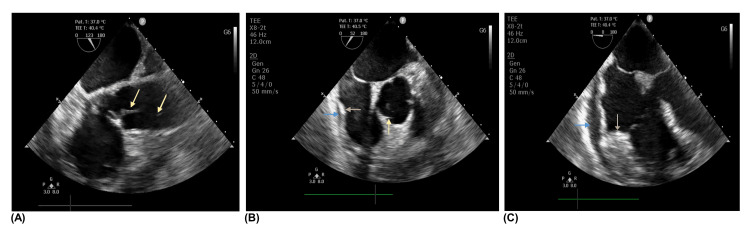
Complementary study during ICU stay - transesophageal echocardiogram. Transesophageal echocardiogram showing large aortic valve vegetation (yellow arrows) (A), moderate pericardial effusion (blue arrows), and echogenic image of moderate dimensions in right atrium-ventricle junction (grey arrows) (B-C). ICU, intensive care unit
